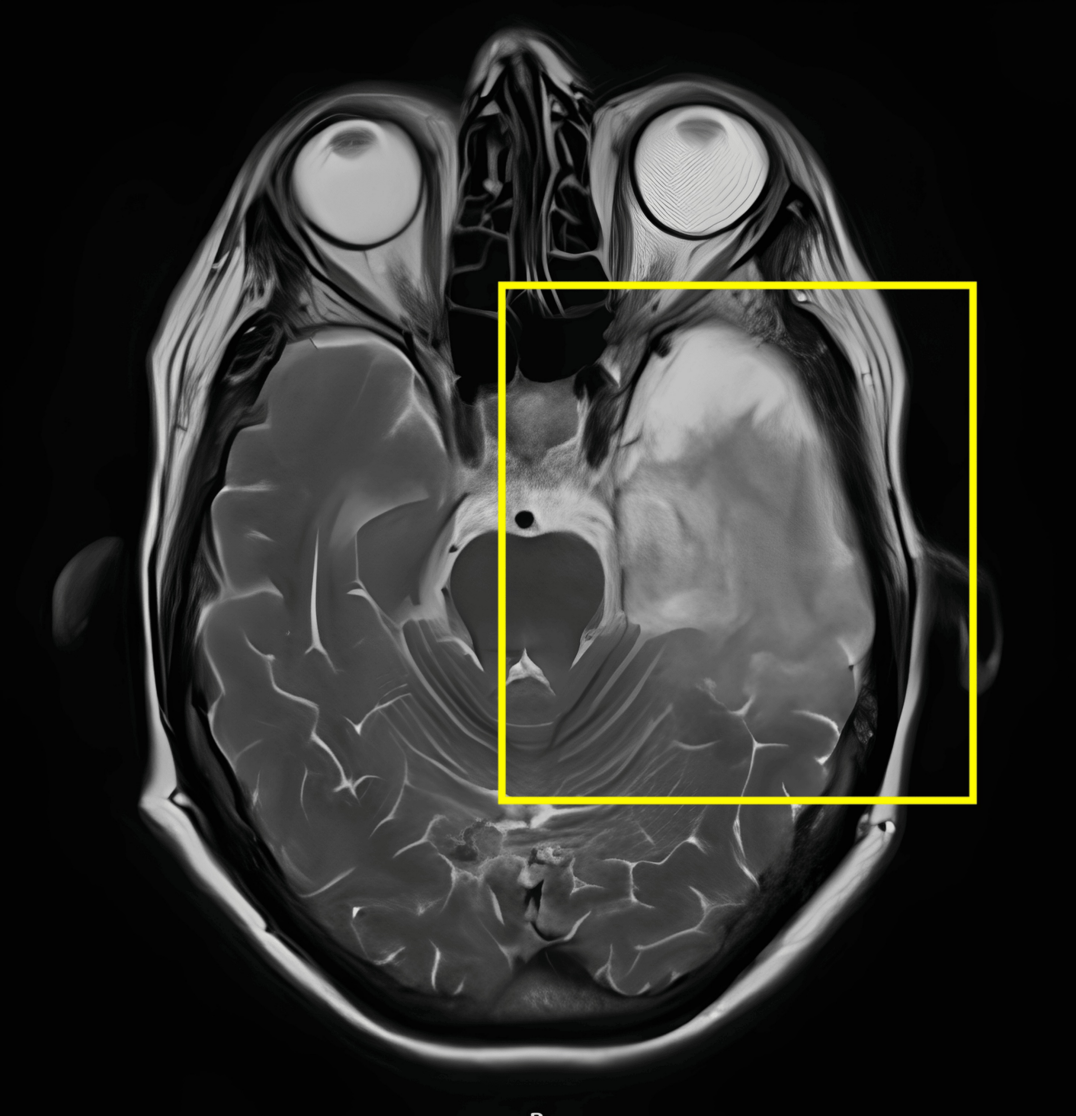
Post-treatment axial T2-weighted MRI Yellow box highlights residual hyperintensity in the right temporal lobe, showing interval improvement after treatment for herpes simplex virus encephalitis.
